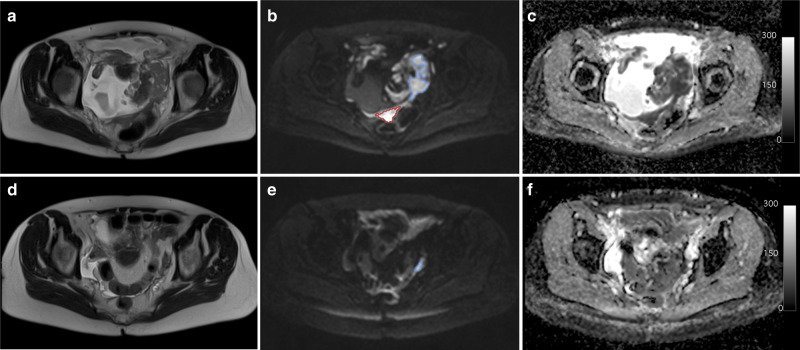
Site-specific response of EOC to neoadjuvant chemotherapy. Images in a 62-year-old woman with stage 3 high-grade serous epithelial ovarian cancer show differential response in primary and metastatic lesions: a axial T_2_-weighted magnetic resonance imaging (MRI) at baseline (pre-NAC), b corresponding axial high-b-value diffusion-weighted MRI (b = 900smm^−2^), c apparent diffusion coefficient (ADC) map and d–f matched sections of the same imaging series after three cycles of platinum-based chemotherapy (post-NAC preoperative). (Scalebar on the ADC map is in units of 10^−5^ mm^2^ s^−1^.) Delineation of regions of interest (ROIs) is shown in b, e for the left ovarian lesion (blue ROI) and peritoneal lesion (red ROI). The ovarian lesion remained measurable on MRI after three cycles of chemotherapy and was included in the imaging-pathology comparison, but the peritoneal lesion was nonmeasurable on MRI after three cycles of chemotherapy. MRI magnetic resonance imaging, NAC neoadjuvant chemotherapy, ADC apparent diffusion coefficient, ROI region of interest.
